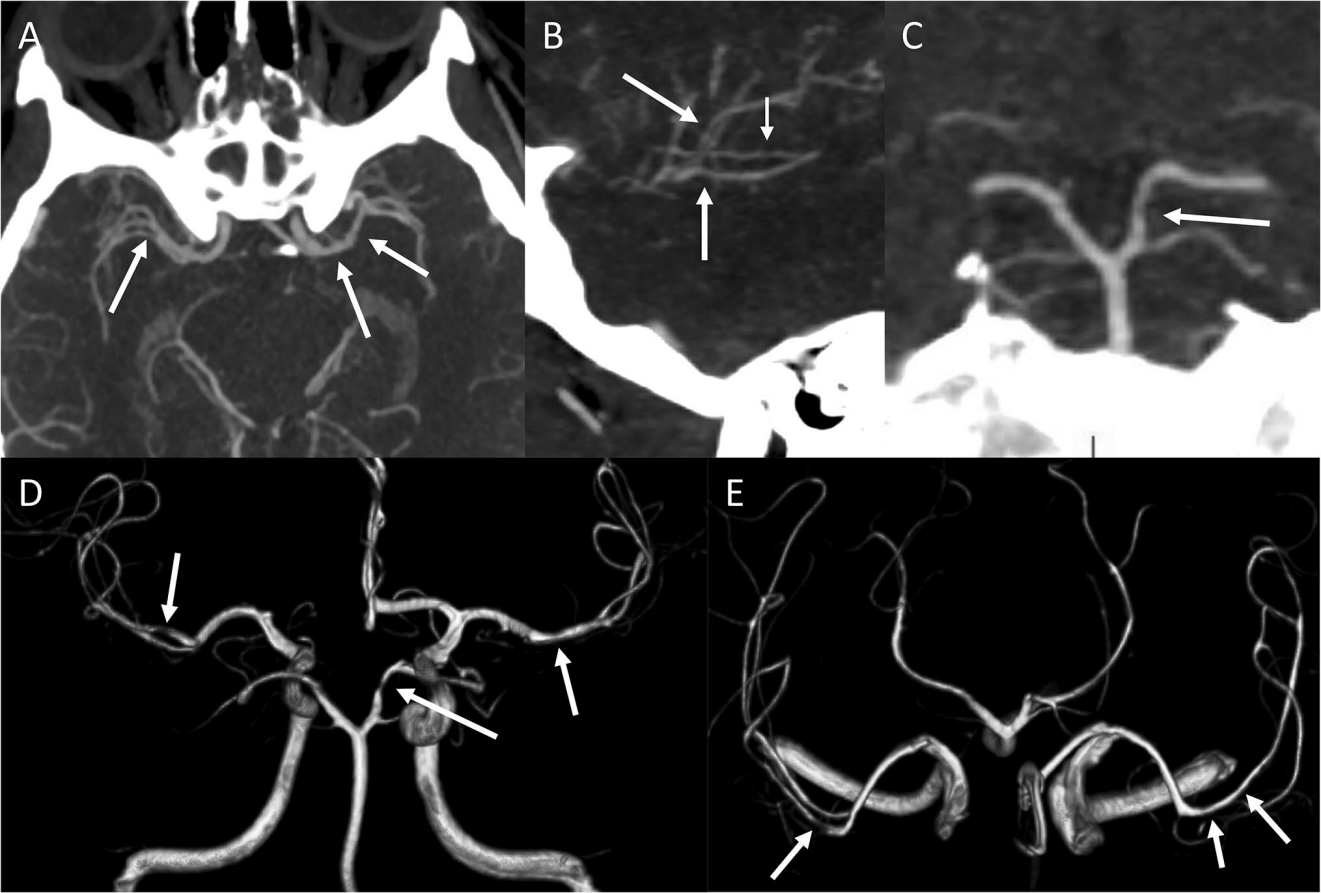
Maximum intensity projections of brain CT angiography at admission demonstrating distinct irregularities of the brain-supplying arteries suspicious of vasospasm. The mainly involved segments were the M1- (A) and M2-segments (B) of both middle cerebral arteries and the P1-segment of the left posterior cerebral artery (C). 3D-time-of-flight-MR angiography on day 5 after admission showing persistent multifocal vasospasms in the M1-segment of both medial cerebral arteries (MCA), the P1-segment of the left posterior cerebral artery (D, coronal) and the M2-segment of both MCAs (E, axial)
